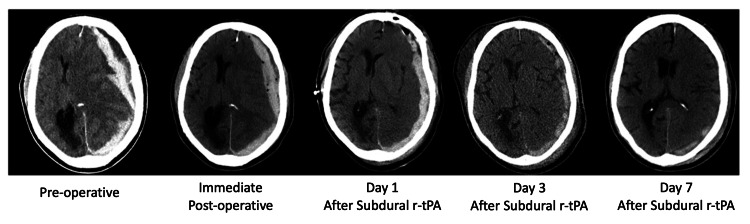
Computed tomography scans Computed tomography scans that show progressive radiologic improvement of the subdural hematoma. The subdural drain was removed on postoperative day 10.
